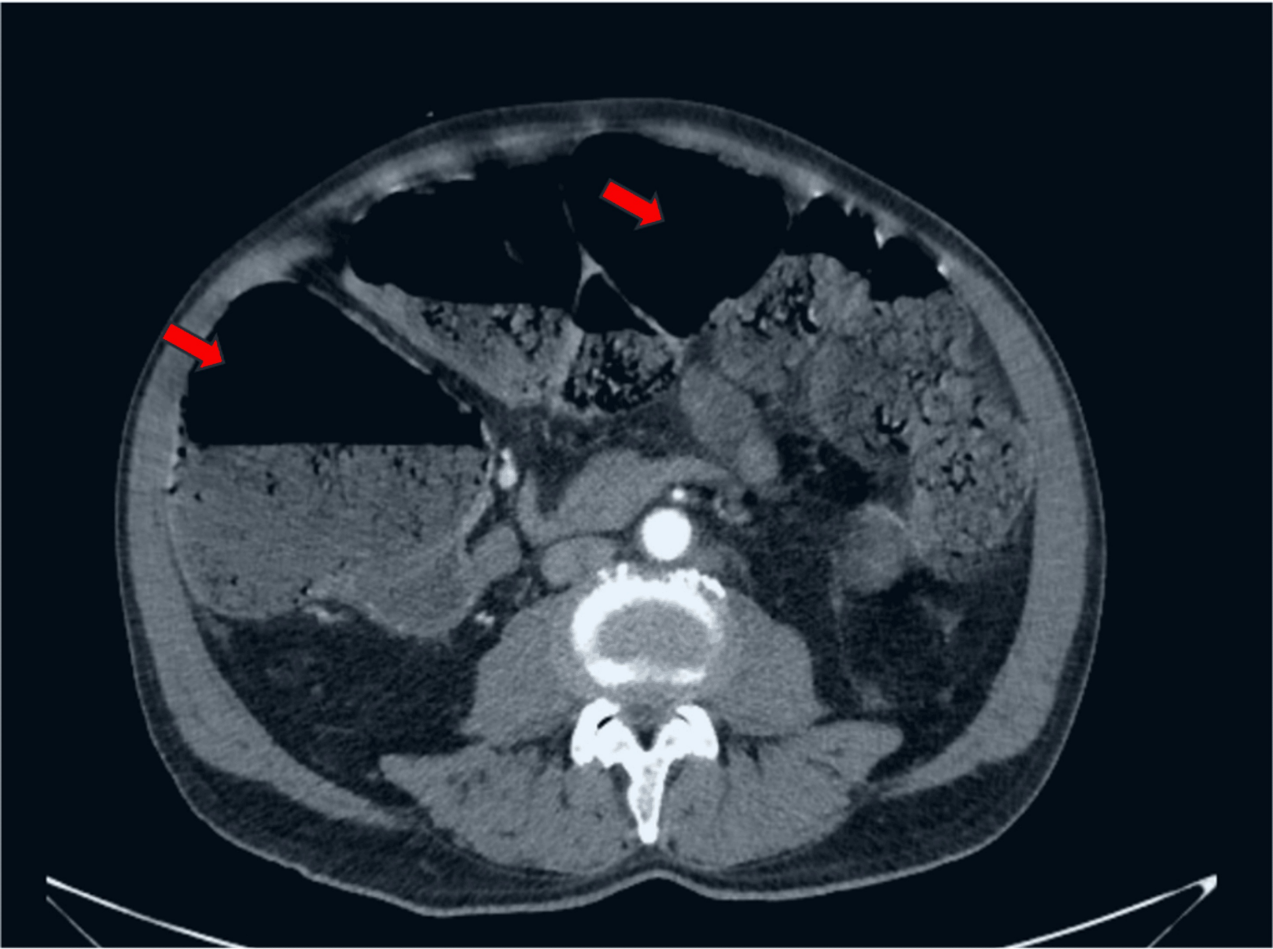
CT scan, axial plane of the abdomen showing bowel dilatation (red arrows)
